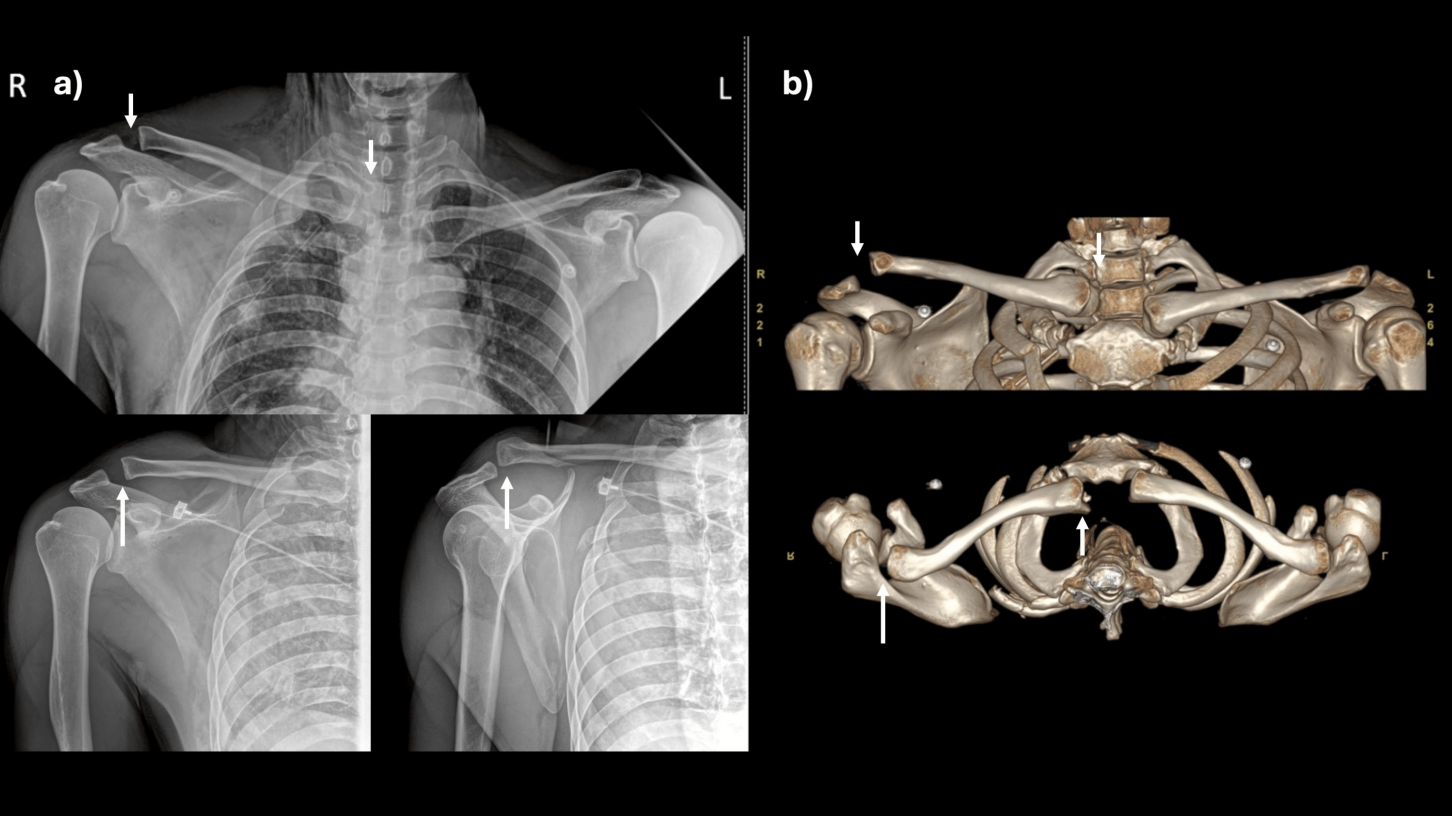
Radiographic examination of the injury. (a) The radiographs of both clavicles, right clavicle anteroposterior view, and transcapular view. (b) The 3D CT scan demonstrated superior displacement of the acromioclavicular joint (arrow) and posterosuperior displacement of the sternoclavicular joint (arrow).
